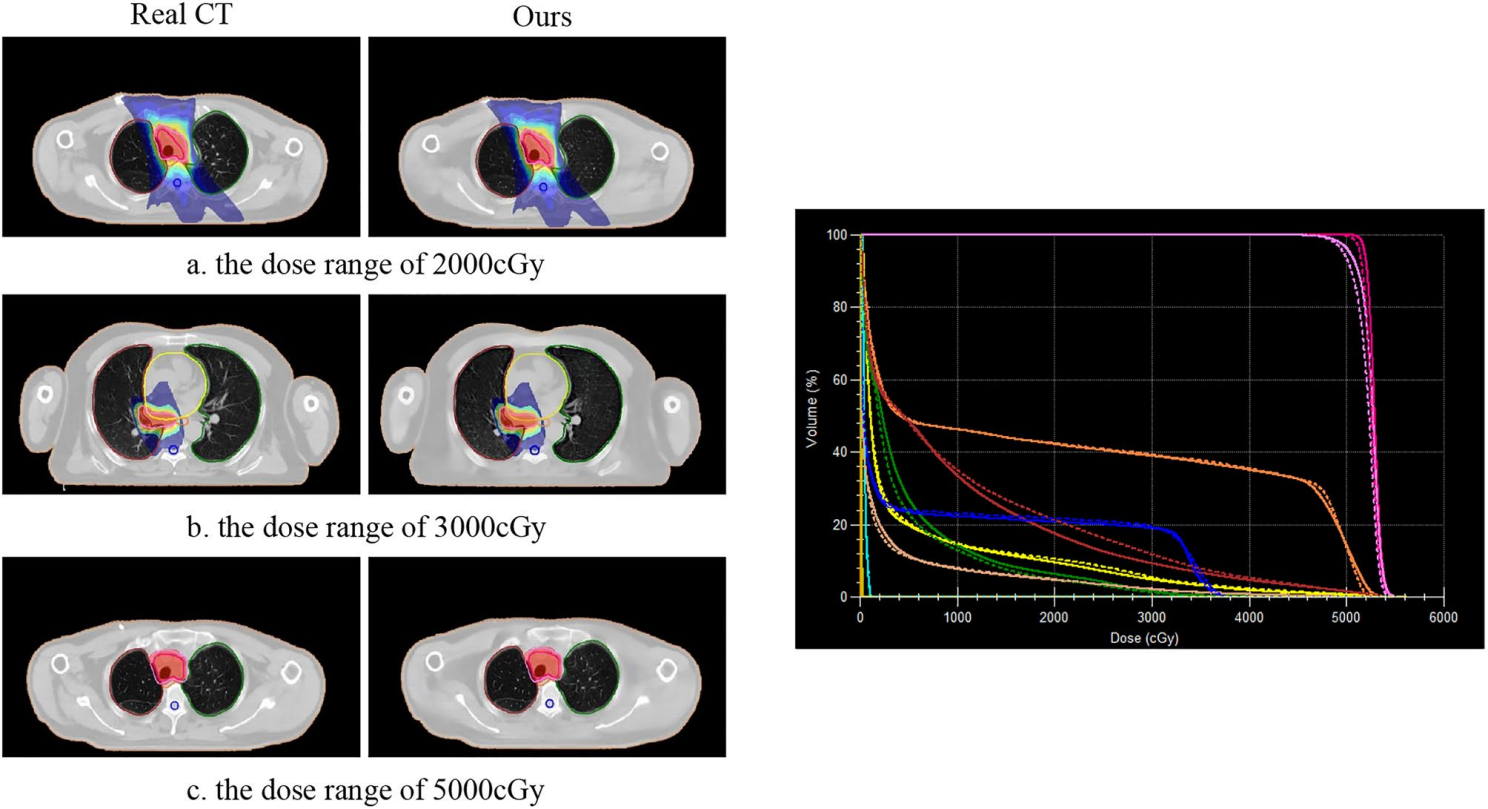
Differences between our method and Real CT for different dose distributions on the Chest dataset (left). Patient DVH differences, where the solid line is Real CT and the dashed line is sCT (right).
